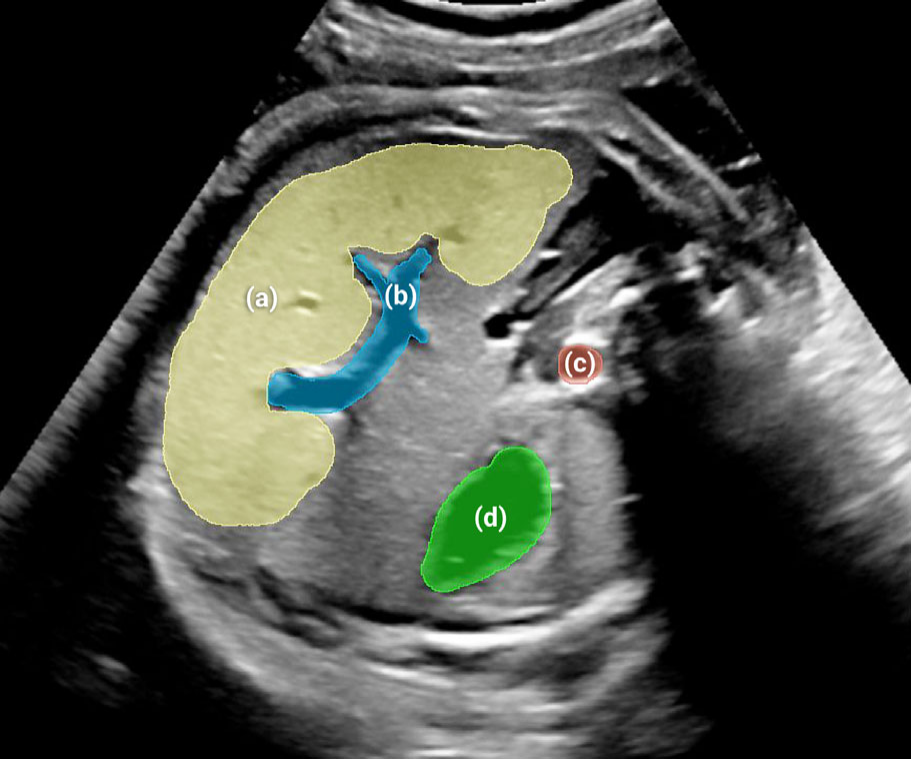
Traditional axial view of fetal abdominal circumference with liver annotation on ultrasound. (A) Liver, (B) intra‐hepatic portion of umbilical vein, (C) aorta, (D) stomach. These structures were annotated together in a parallel study about automated semantic segmentation and object detection, for which the dataset is available.^20^
